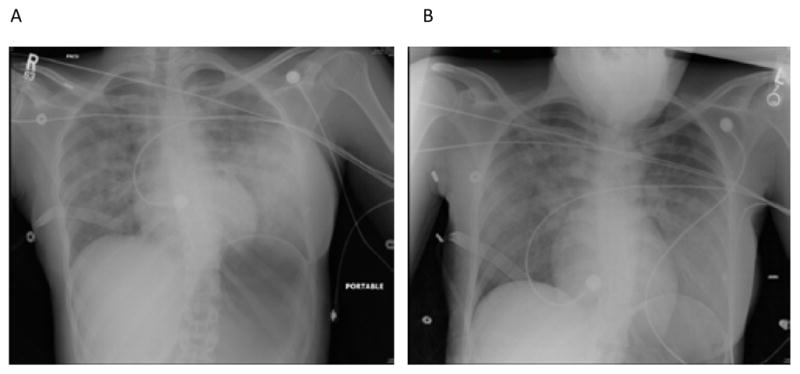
Postoperative chest x-rays. Figure 1A is the chest X-ray performed 1 hour after surgery in the PACU. Moderate pulmonary edema is demonstrated. Figure 1B is the chest X-ray obtained in the morning of postoperative day 1. Improving pulmonary edema, likely hydrostatic, is demonstrated.
